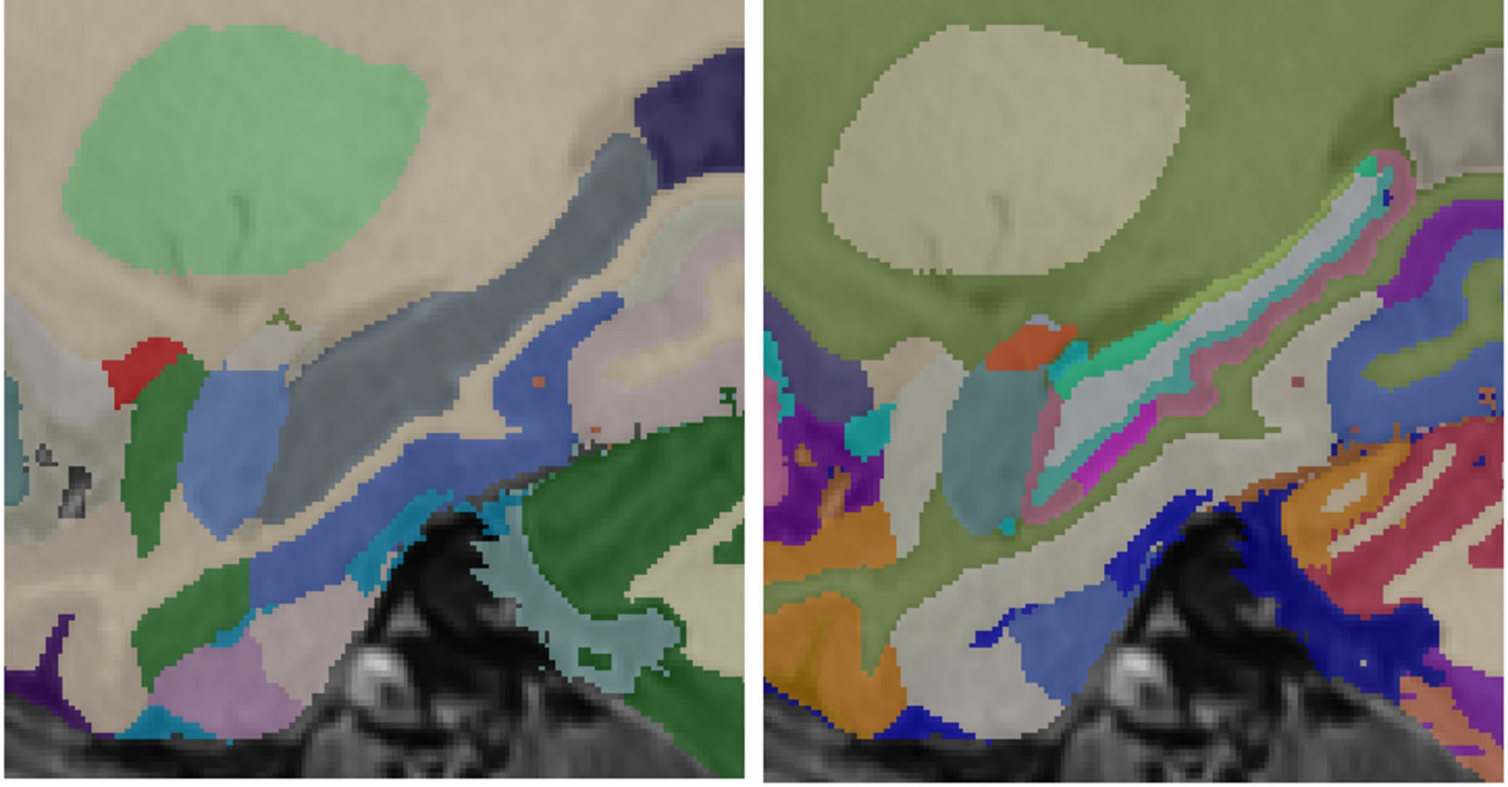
Example of the old and new hippocampus subfield definition on top of a reference T1w MRI.
